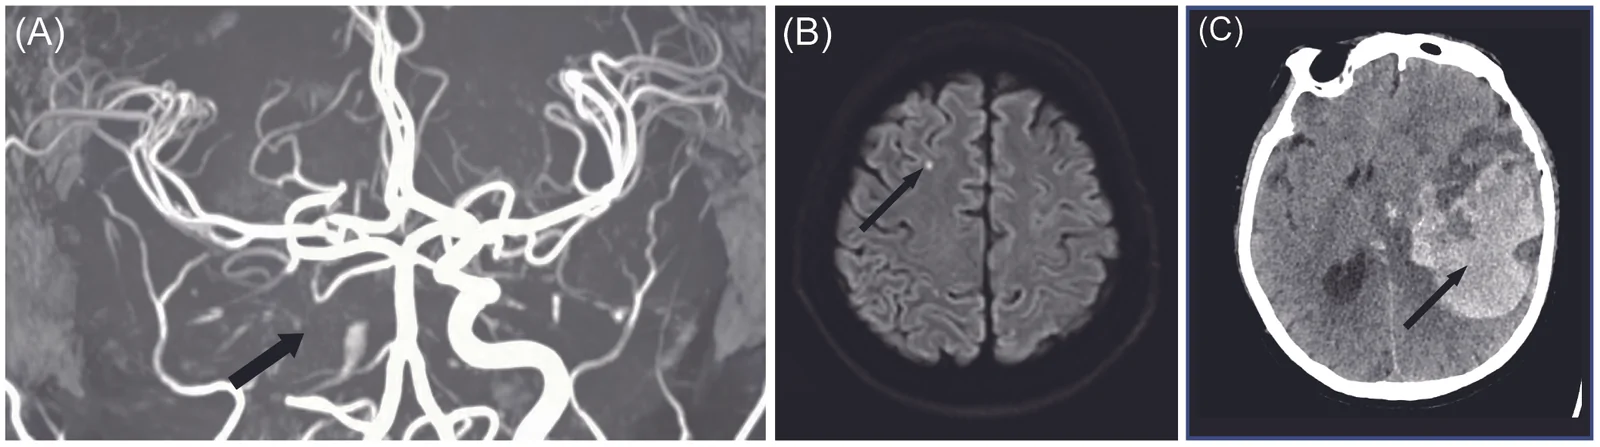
Representative neuroimaging findings corresponding to key components of the angioinvasion severity score. (A) Magnetic resonance angiography (MRA) showing occlusion of the internal carotid artery (arrow). (B) Diffusion-weighted imaging (DWI) demonstrating acute cerebral infarction (arrow). (C) Non-contrast computed tomography (CT) demonstrating intracranial hemorrhage (arrow).
